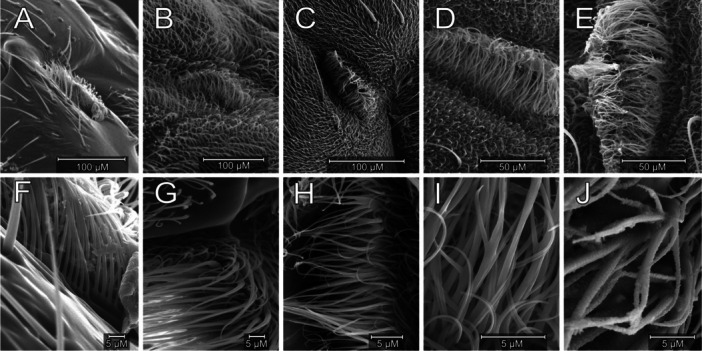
Variation in anterior thoracic spiracle (AS) microtrichia. Upper and lower rows represent paired images of the same species at lower (450×) and higher (10,000–20,000×) magnification, showing uniform, organized microtrichia (A, F—*Mosillus bidentatus*), nonuniform, organized microtrichia (B, G—*Parydra aquila*), and varying degrees of unorganized, nonuniform dendritic microtrichia (C, H—*Paracoenia bisetosa* and D, I—*Paracoenia turbida*). *Ephydra hians* (E, J) shows the most highly dendritic, least uniform microtrichia. Surface texture in J persisted after ultrasonic cleaning.
